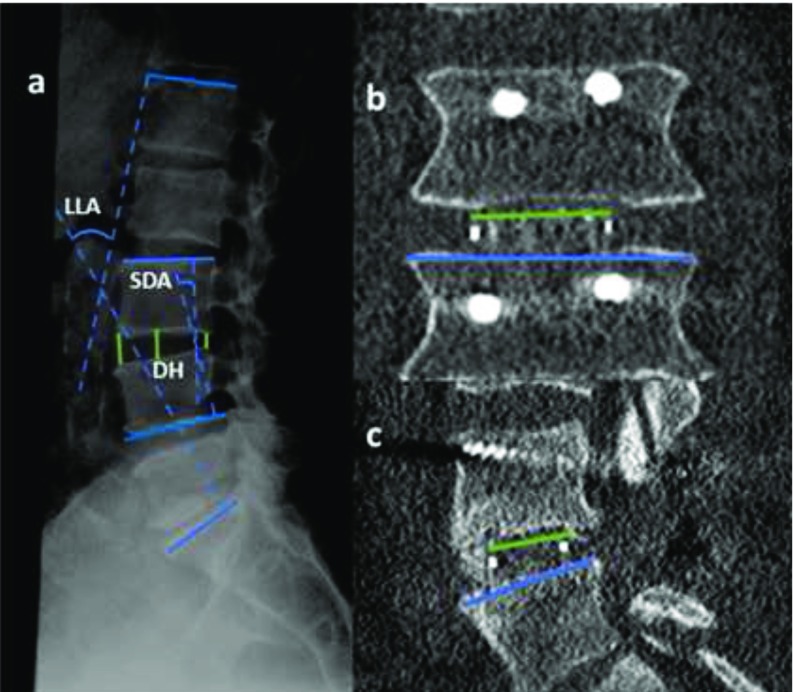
(a) Measurement of LLA, SDA and A,M,P/DH using lateral plain X-ray in a neutral position, (b,c) % CC along transverse and anteroposterior diameters.
